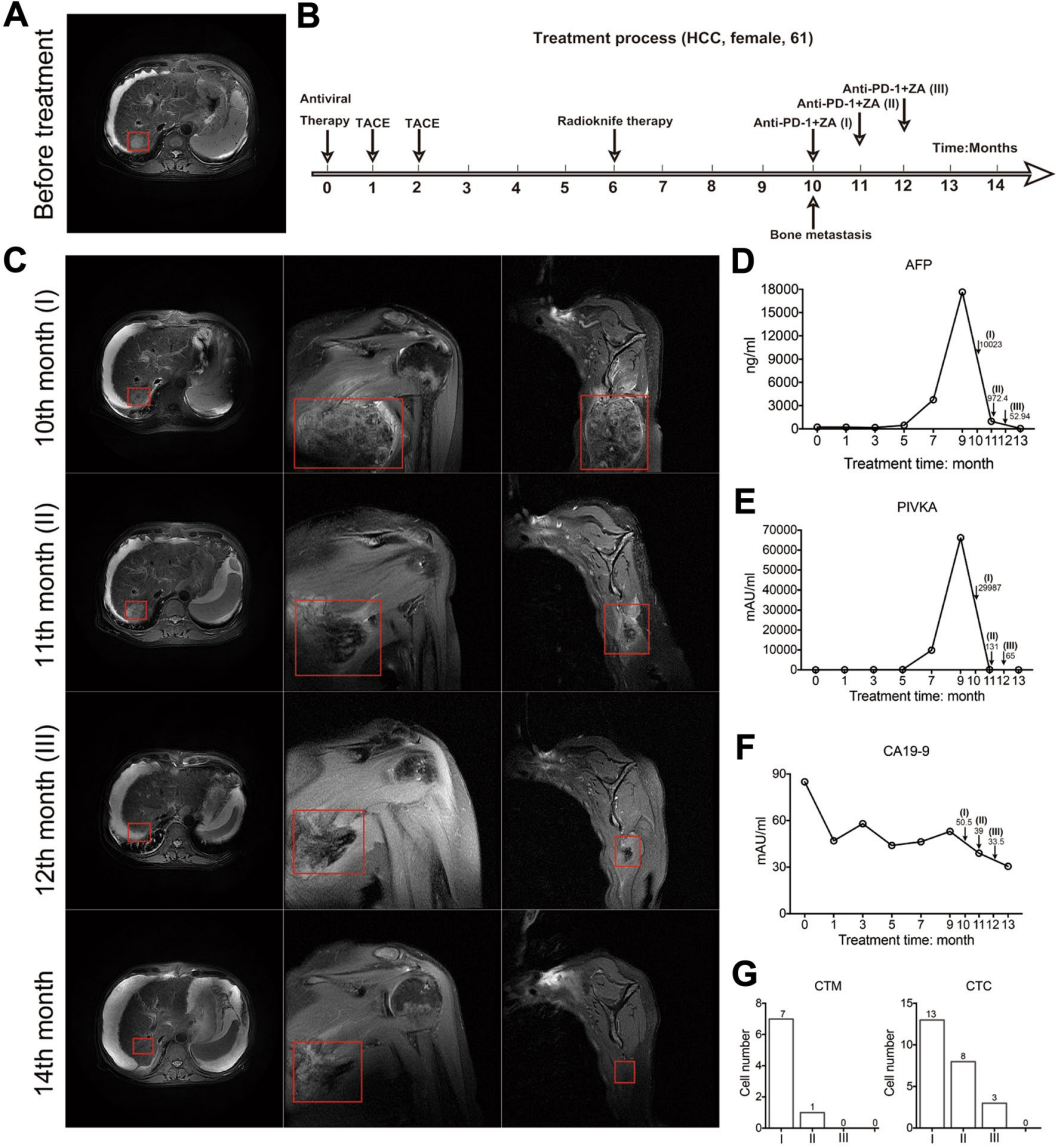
The case of combined ZA and anti-PD-1 in the treatment of bone metastasis from liver cancer. A MRI of the primary lesion of the liver cancer before treatment. B The process of treatment. (Antiviral therapy was given within the first month after the diagnosis of primary liver cancer.) The patient received two TACE treatments in the first and second month. At the 6th month, radioknife treatment was used for the patient. Bone metastasis was found at the 10th month. The anti-PD-1 treatments combined with ZA were applied in the 10th, 11th and 12th months, respectively. C MRI images of the liver and left scapula after the patient was found to have bone metastasis. At the 11th month, the left scapular lesion was significantly reduced on the 7th day after treatment. At the 12th month, the lesion in the right lobe of the liver was stable, and the metastasis in the left scapula was significantly reduced. At the 14th month, there was no progression of the intrahepatic lesion, and the extrahepatic metastasis almost disappeared. D, E High AFP and PIVKA were detected and subsequently reaching maximum values when bone metastasis was observed. Both of them decreased gradually after anti-PD-1 combined ZA treatment. F The patient had a high level of CA19-9 at diagnosis. From the beginning to the tenth month, the level of CA19-9 gradually decreased but remained above normal. And the rate of decrease gradually slowed down or even slightly increased. After the third administration of anti-PD-1 + ZA, CA19-9 decreased to the normal range (< 37mAU/ml). G With the application of the combined treatment, CTC and CTM were gradually reduced to 0
